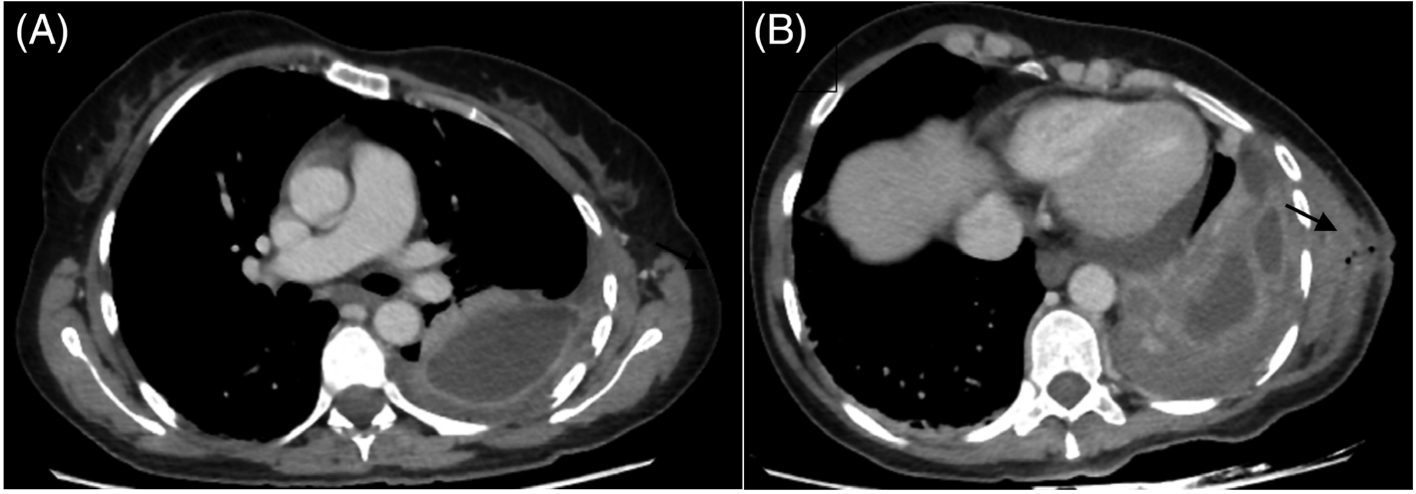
(A) Contrast thoracic CT axial cuts in mediastinum and lung windows showing the left pleural effusion with thickening of the pleura and split pleura sign. (B) Contrast thoracic CT axial cuts in mediastinum and lung windows showing the pleural fluid extended through a chest wall wound (wound opening—black arrow)
